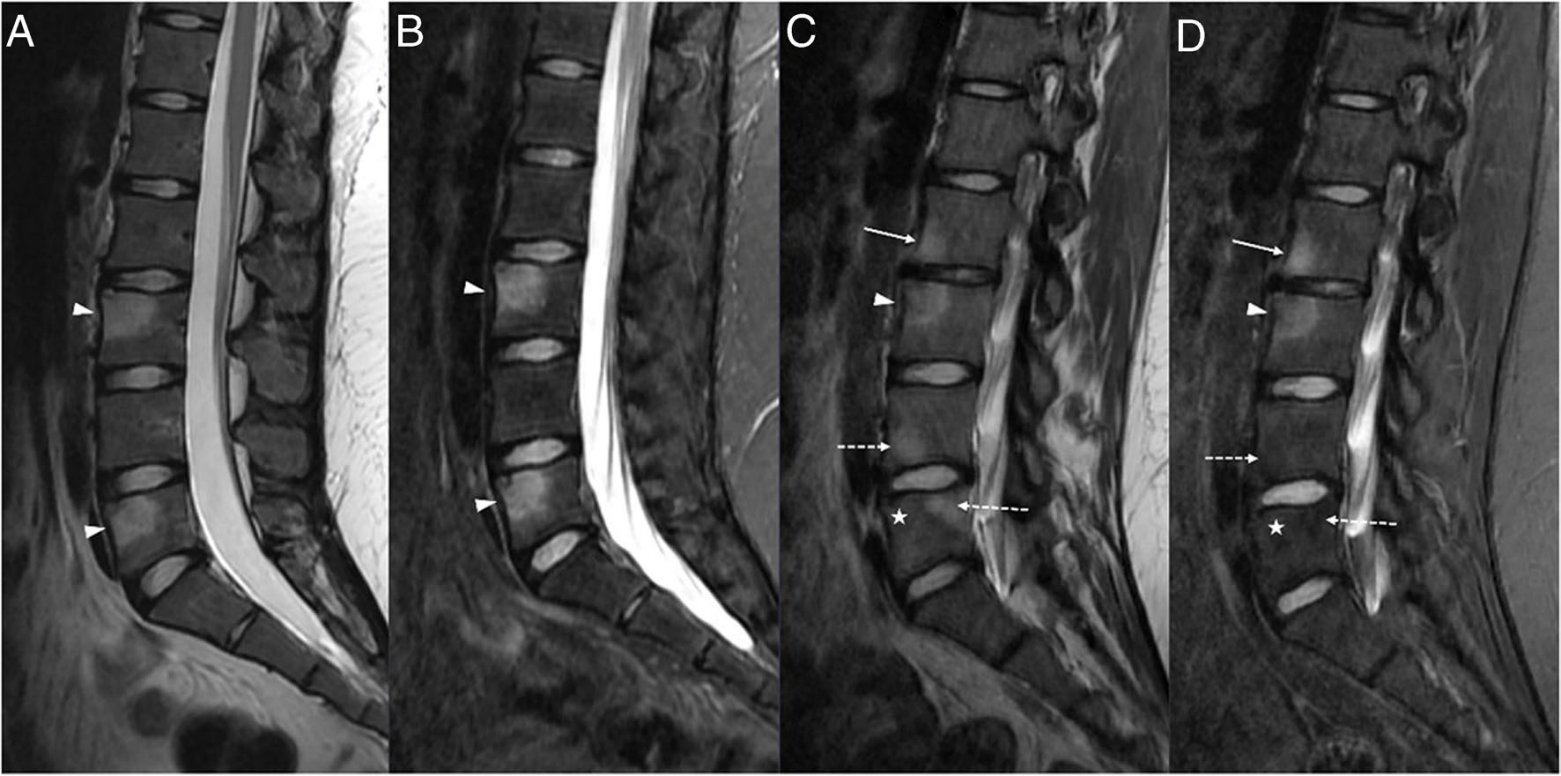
Evolution of vertebral lesions and recurrence: magnetic resonance images of the lumbar spine obtained from a girl at ages 15 (a, b) and 17 (c, d) years. a, b Sagittal T2-weighted (a) and sagittal T2-weighted fat-saturated (b) images show bone edema in the bodies of lumbar (L) vertebra 3 and L5 (*arrowheads*). c, d Sagittal T2-weighted (c) and sagittal T2-weighted fat-saturated (d) images of the same patient about 2 years later show a new inflammatory lesion with bone edema in L2 (*arrow*), as well as partial resolution of the previous L3 lesion (*arrowhead*), complete resolution of the previous L5 lesion (*asterisk*), and areas of fat replacement in L4 and L5 representing sequelae of resolved inflammatory lesions that appeared in the time between the two exams (*broken arrows*)
